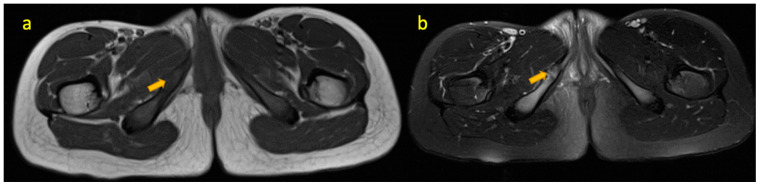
(a,b) Axial T1W and axial T2W FS MR images of an 11-year-old girl showing mild bone marrow oedema signal around mildly enlarged right ischiopubic synchondrosis consistent with osteochondrosis. There is no surrounding soft tissue collection.
